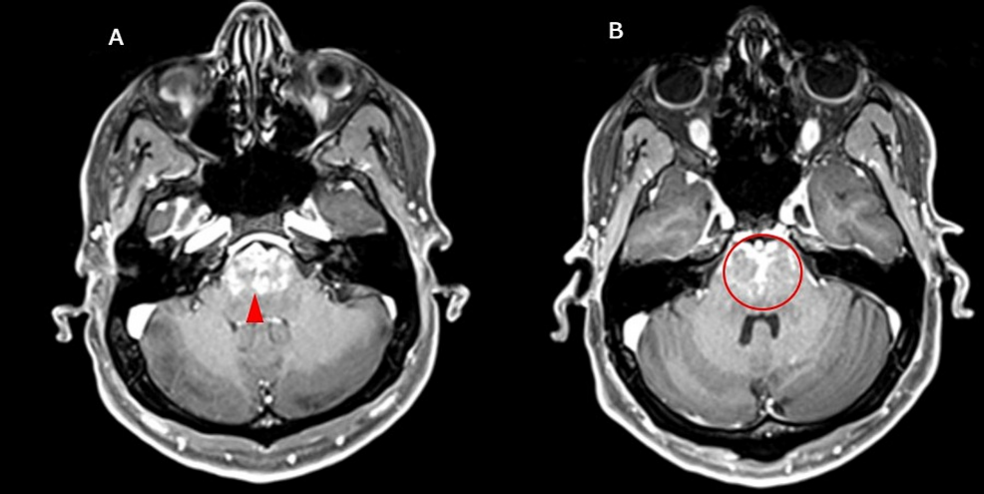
Axial brain T1-weighted MRI with contrast showing (A) hyperintense signal (arrowhead) involving the pons and (B) heterogeneous enhancement (encircled) in the pons on the post-contrast study. MRI: magnetic resonance imaging
